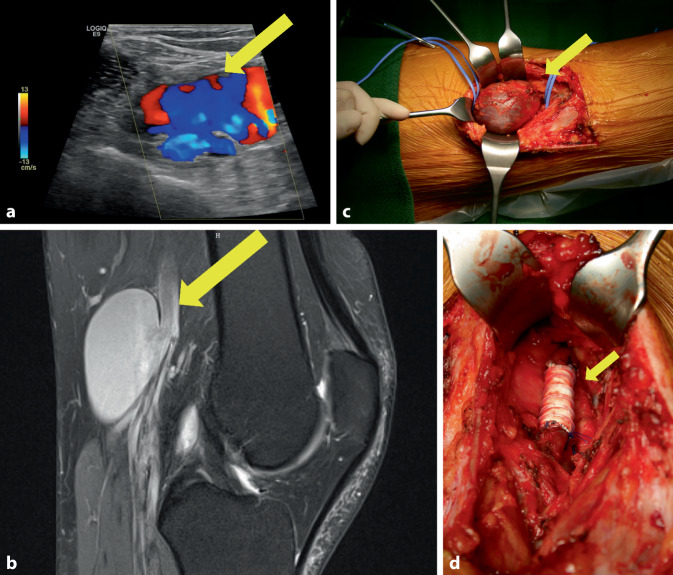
Fall 2: Venöses Poplitealaneurysma links. a Darstellung des Aneurysmas der V. poplitea links in der farbkodierten Duplexsonographie (*gelber Pfeil*), b Darstellung des venösen Aneurysmas in der MR-Angiographie in sagittaler Schnittrichtung (*gelber Pfeil*), c Intraoperative Darstellung des venösen Poplitealaneurysmas während der Primäroperation (*gelber Pfeil*), d Intraoperative Darstellung der rekonstruierten V. poplitea mittels GORE-TEX®Vascular Graft-Prothese (W.L. Gore, Putzbrunn, Deutschland) auf einer Länge von ca. 4 cm (*gelber Pfeil*)
